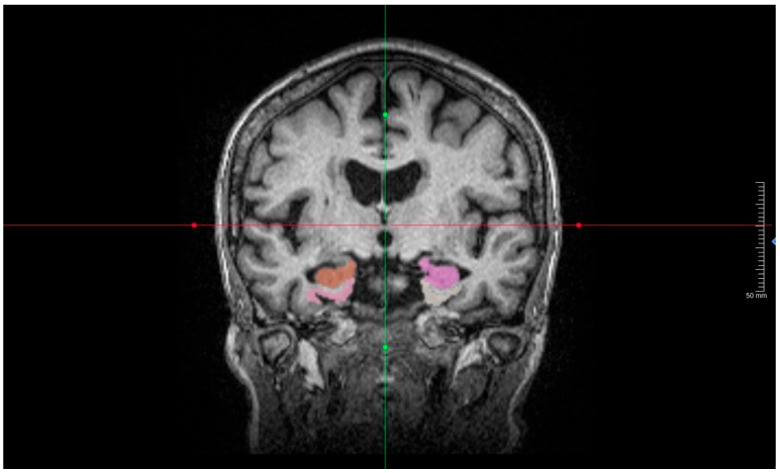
Detailed visualization of medial temporal lobe segmentation. Coronal 3D T1-weighted MRI slice demonstrating uAI^®^-derived segmentation of Alzheimer’s disease-relevant medial temporal structures. Colored overlays represent automatically segmented regions used for volumetric calculations (e.g., hippocampus and adjacent medial temporal cortex). The image is intentionally magnified to highlight the medial temporal structures, and the intersecting lines indicate the navigational axes.
